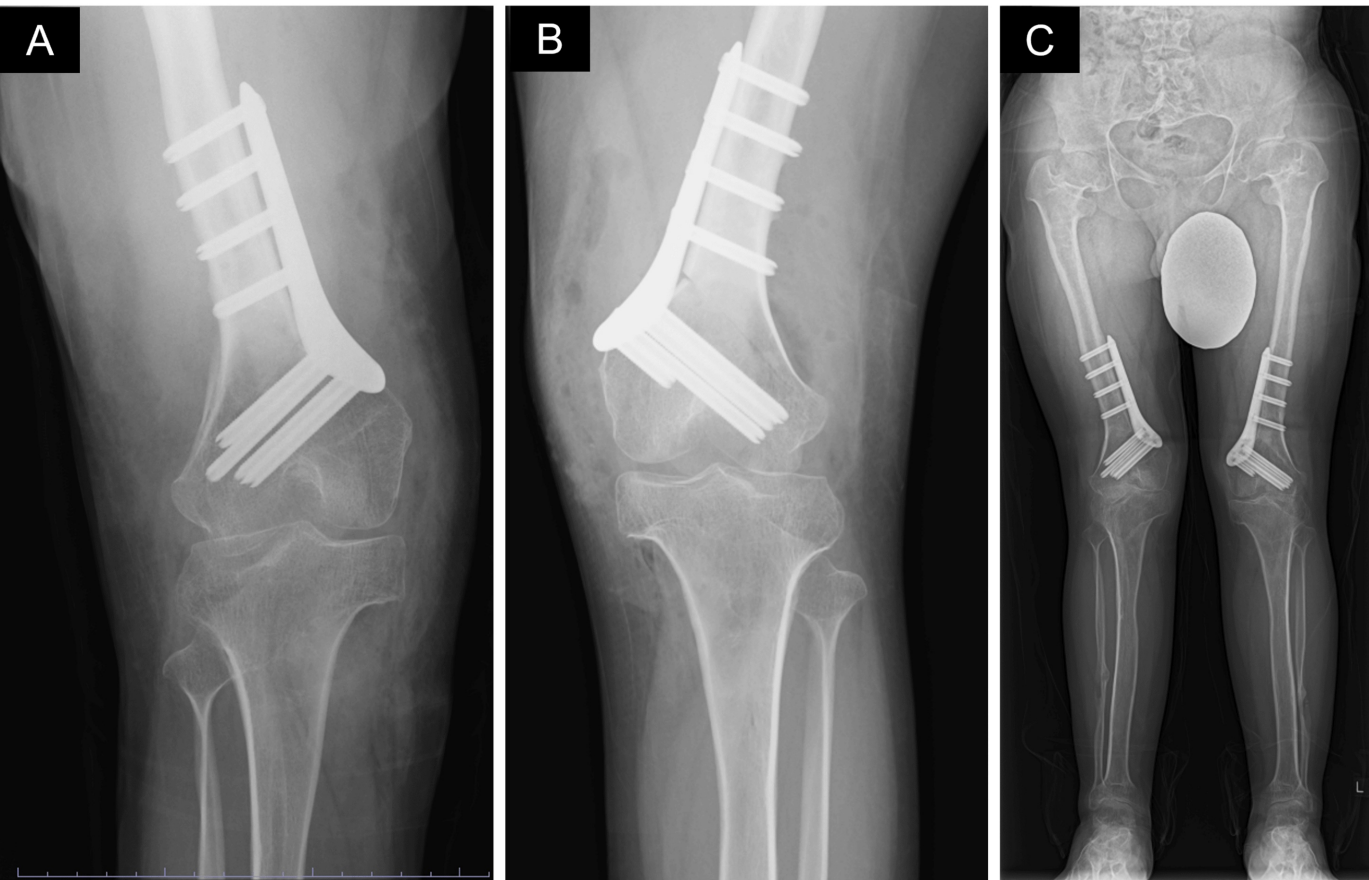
Postoperative X-ray images A) Right knee immediately after the operation; B) Left knee immediately after the operation; C) Standing posture four months after right knee surgery.
